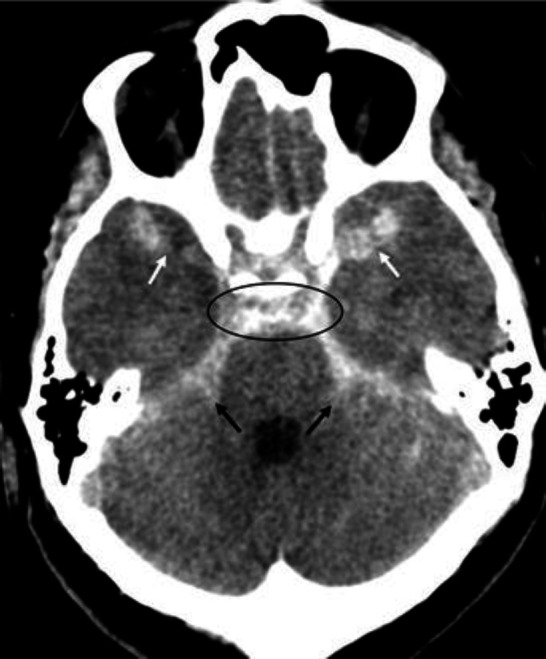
Non-contrast CT brain of a 51 year-old female with a sub-arachnoid haemorrhage. The hyperdense areas seen in the prepontine cistern (circled), cerebellopontine angle cistern (black arrows) and sylvian fissures (white arrows) represent acute haemorrhage
